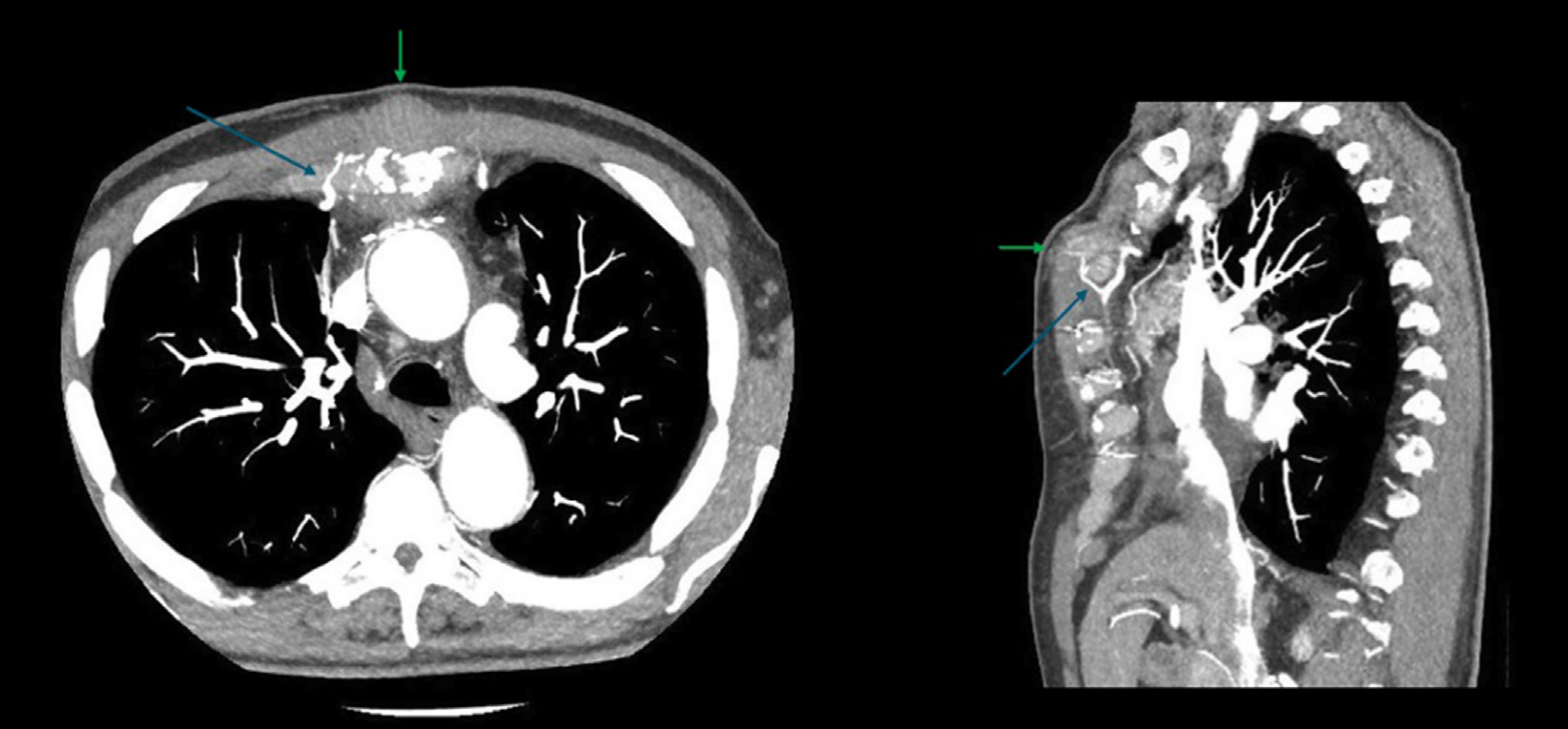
Enhanced CT Angiogram Enhanced CT angiogram in the arterial phase with maximum intensity projection (MIP) of axial (right) and sagittal (left) images, demonstrating a small feeding vessel from the right IMA (blue arrow), supplying the pseudoaneurysm sac in the midline anterior to the sternum (green arrow). CT = computed tomography; IMA = internal mammary artery.
